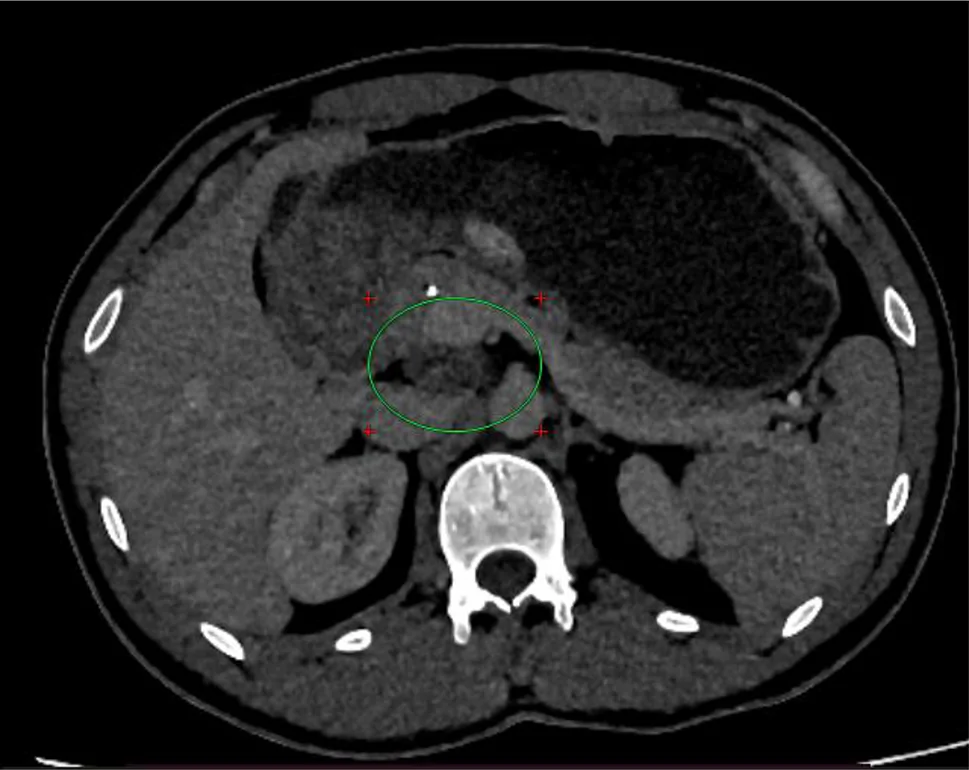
Contrast enhanced CT scan of the abdomen showed significant reduction of the lesion
